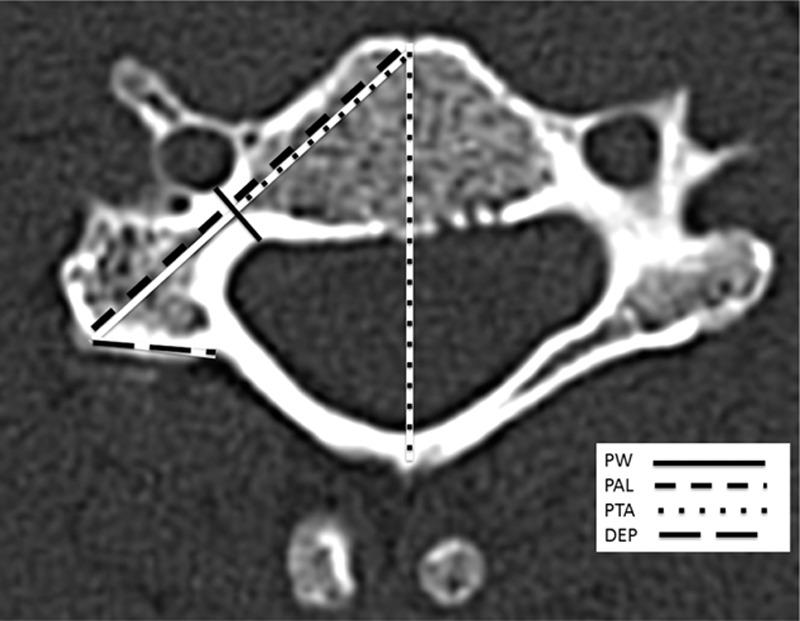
Axial computed tomography reconstruction.
